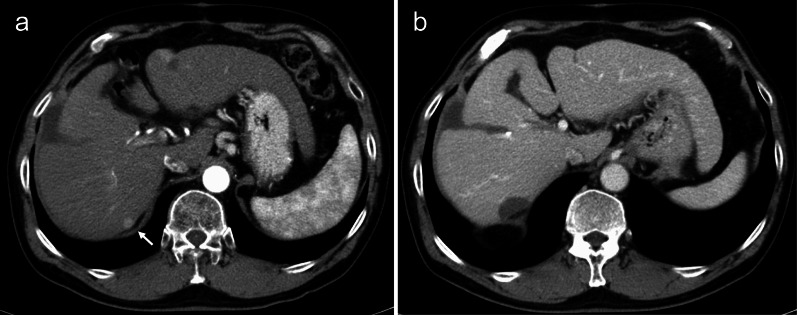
a CT image of hepatocellular carcinoma at segment 6/7. It was well enhanced in the arterial phase (arrow). b CT image of post-RFA for hepatocellular carcinoma at segment 6/7
